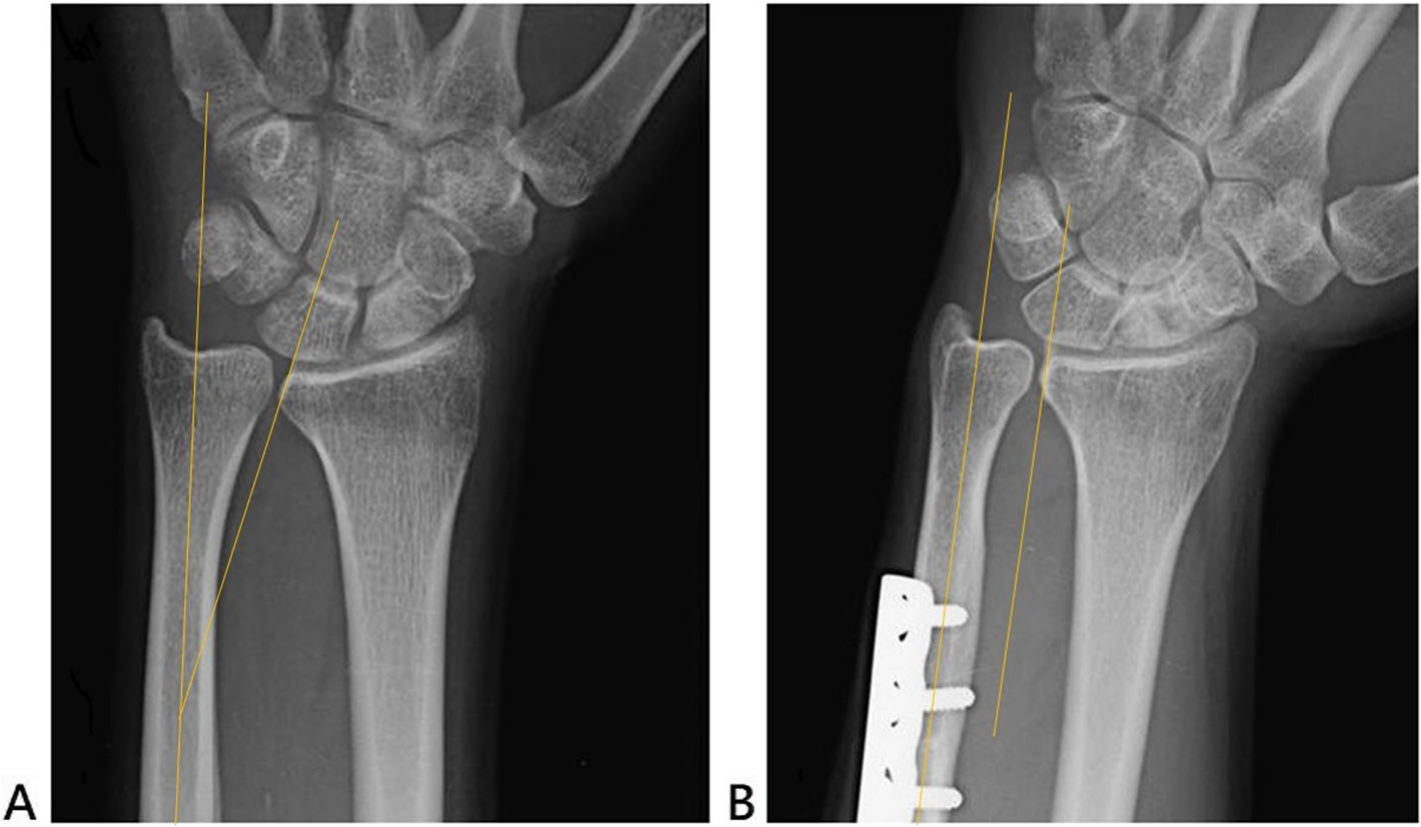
A 27-year-old male patient. a. Radiograph of the left wrist before USO showing the reverse oblique sigmoid notch; b. Radiograph at the 36-month follow-up after surgery. The bony inclination of the sigmoid notch had become more parallel
